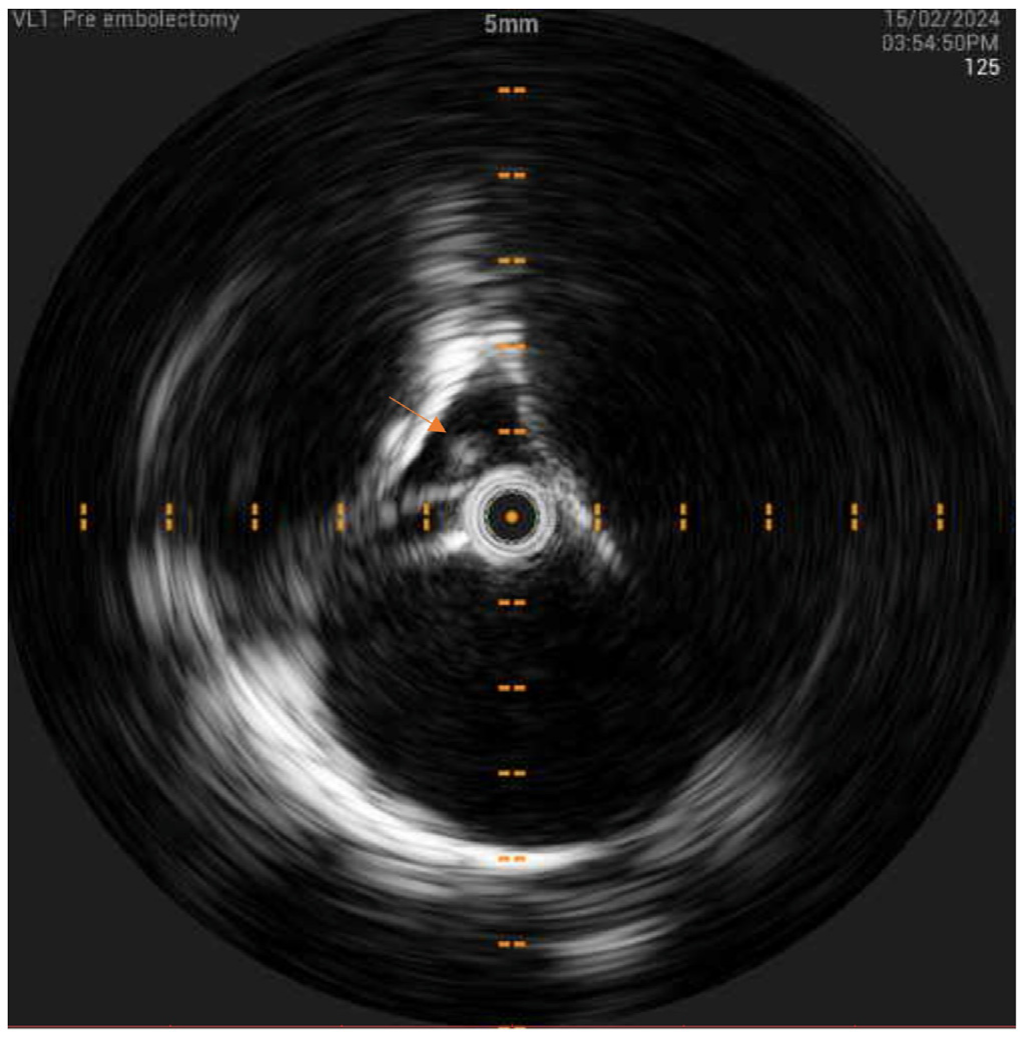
Intravascular ultrasound demonstrating thrombotic material in the right atrium demonstrated with a *superimposed arrow*.
